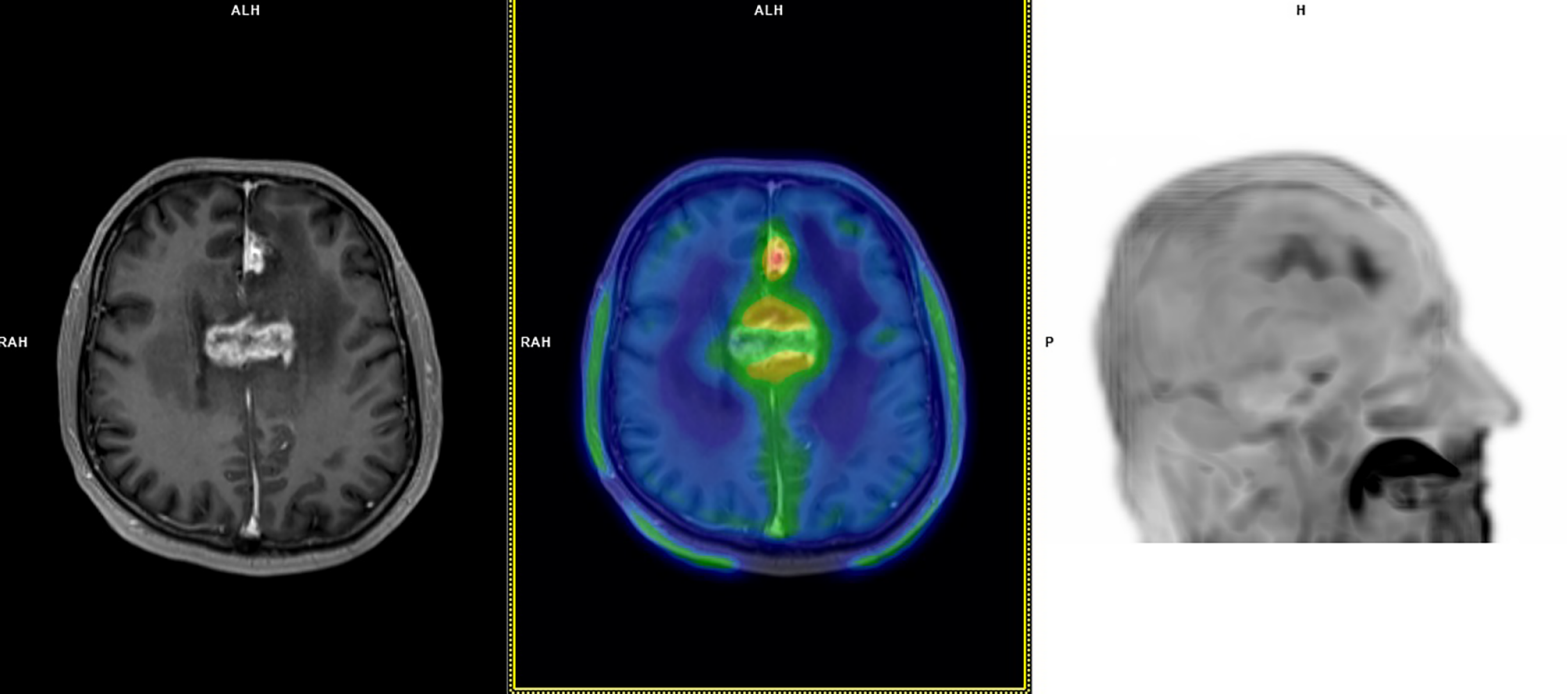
A 60-year-old patient with bioptic diagnosis of astrocytoma WHO grade 3 with lesions in the left frontal lobe and corpus callosum (IDH wild-type; MGMT methylated) treated with chemoradiation (completed in March 2018) and re-irradiation in July 2018. Follow-up MRI scans in October 2018 documented an increasing T2/FLAIR signal and irregular contrast-enhancement at the medial aspect of the left frontal lobe and corpus callosum; no diffusion restriction was seen, and perfusion weighted imaging demonstrated slight increase in Ktrans e Ve values without abnormal Vp. MRI appearances were deemed dubious for GR, favouring the hypothesis of TICs. ^18^F-FET PET performed in November 2018 demonstrated focal tracer uptake at late static imaging (TBRmax: 3.4), a TAC pattern with a TTP at 20 min and subsequent plateau (TAC pattern 2). ^18^F-FET PET was considered suggestive of GR. MRI performed in January 2019 documented GR, and chemotherapy was started. This patient died in July 2019 (PFS: 1 month; OS: 8 months).
